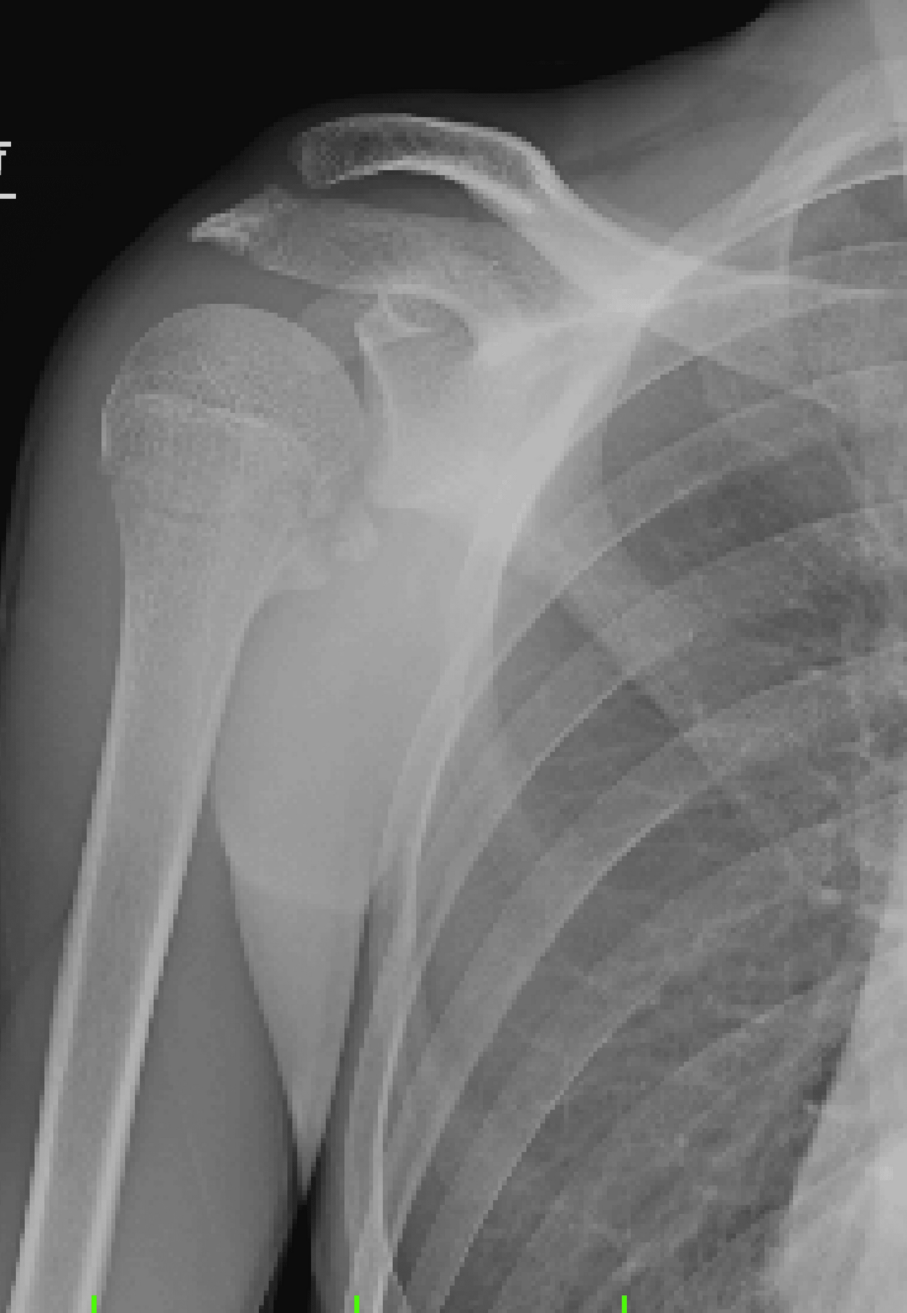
Plain radiography on the internal rotation view
